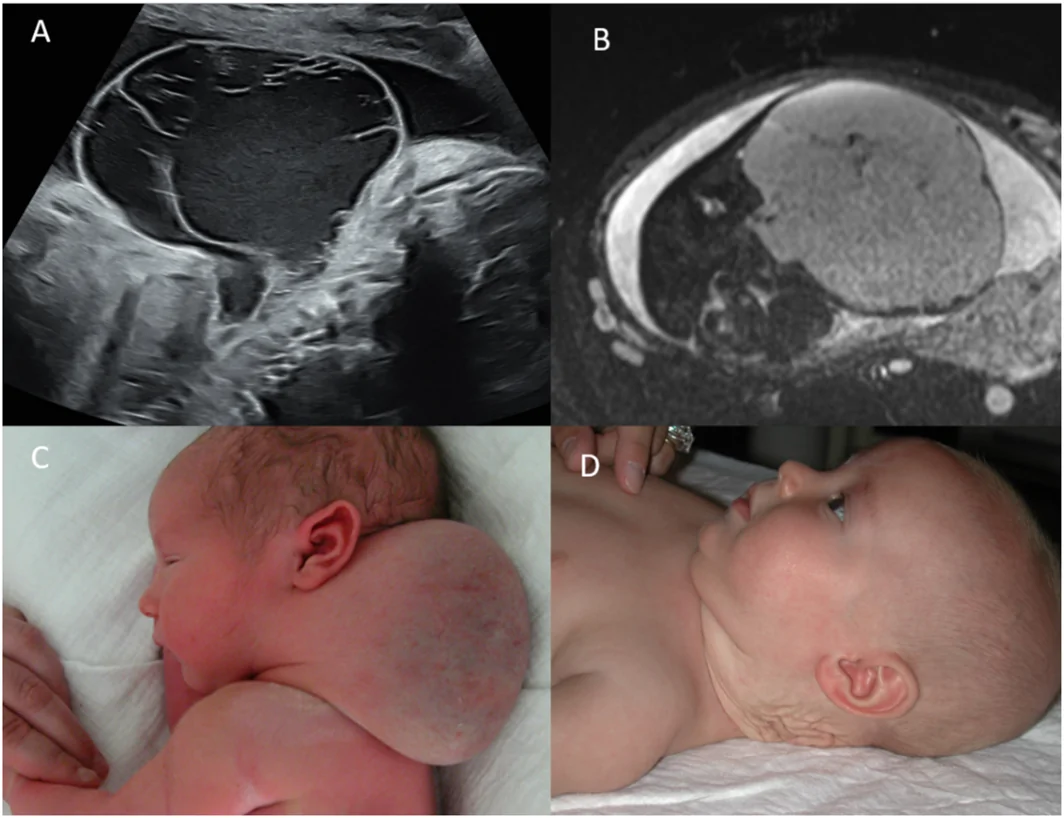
(A‐D): Patient referred for extensive cervico‐facial cystic lymphatic malformation. Prenatal ultrasound at 28 weeks' gestation demonstrated a large cervicofacial cystic lymphatic malformation. Fetal MRI performed at 29 weeks' gestation showed a predominantly pedunculated lesion without deep cervical extension. Based on these imaging findings, maternal sirolimus therapy was not indicated and postnatal sclerotherapy was planned. Postnatal clinical appearance at birth and clinical evolution 4 months after sclerotherapy performed in our referral center showed a favorable outcome.
